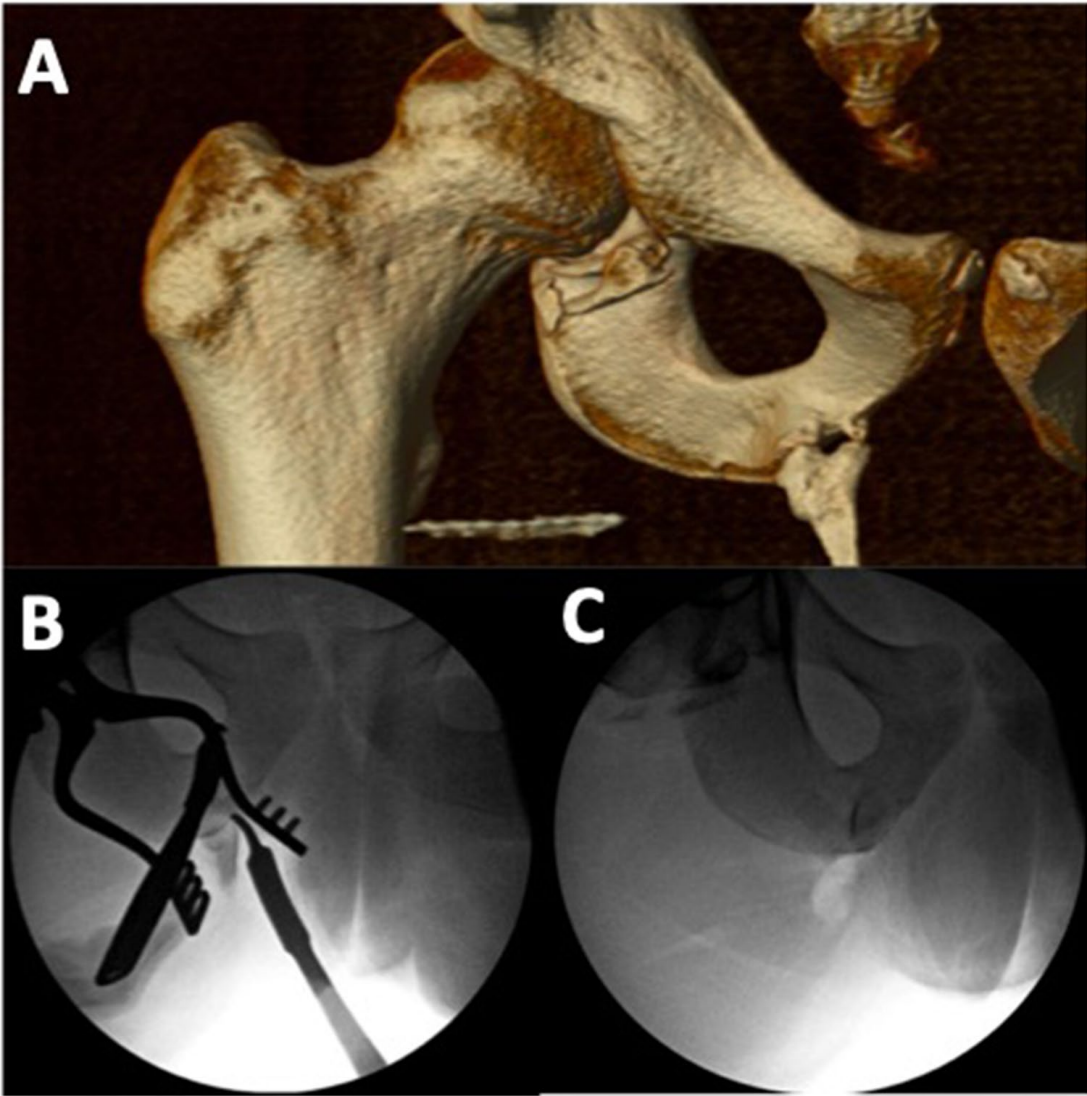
A Computed tomography 3D reconstructions and B intra-operative fluoroscopy demonstrating heterotopic ossification of the right femur and ischium in a 16-year male who suffered severe traumatic brain injury from a motor vehicle accident. C Intra-operative fluoroscopy demonstrating resection of the prior ischial heterotopic ossification
